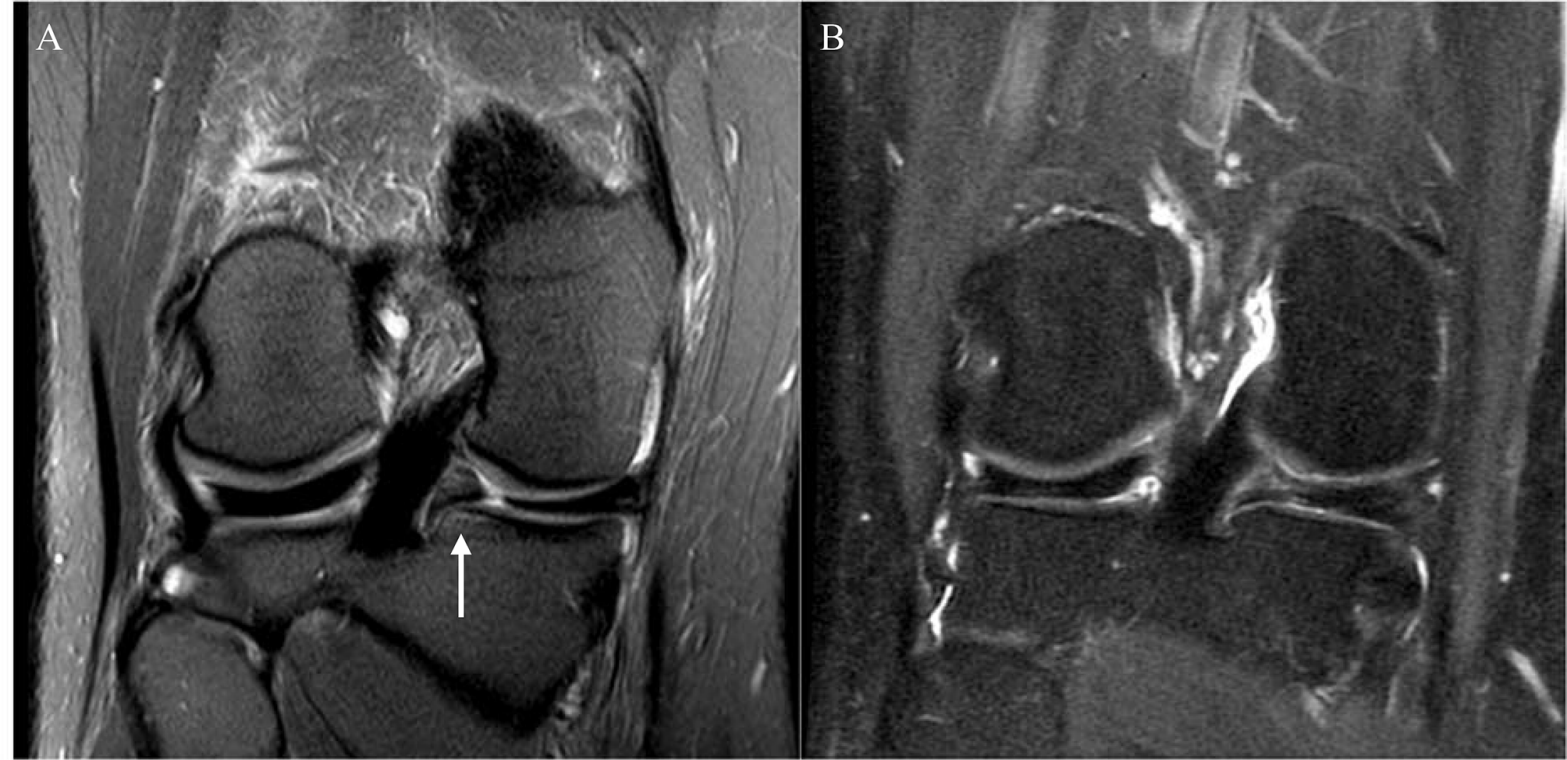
A 45-year-old woman presented with left knee pain and swelling for three days. (A) Baseline MRI showed a radial tear at the posterior root of the medial meniscus with associated bone marrow edema-like lesion at the meniscal root attachment (arrow). (B) At three years after the arthroscopic meniscal root repair. The meniscal root showed partial healing with no longer seen associated bone marrow edema-like lesion
